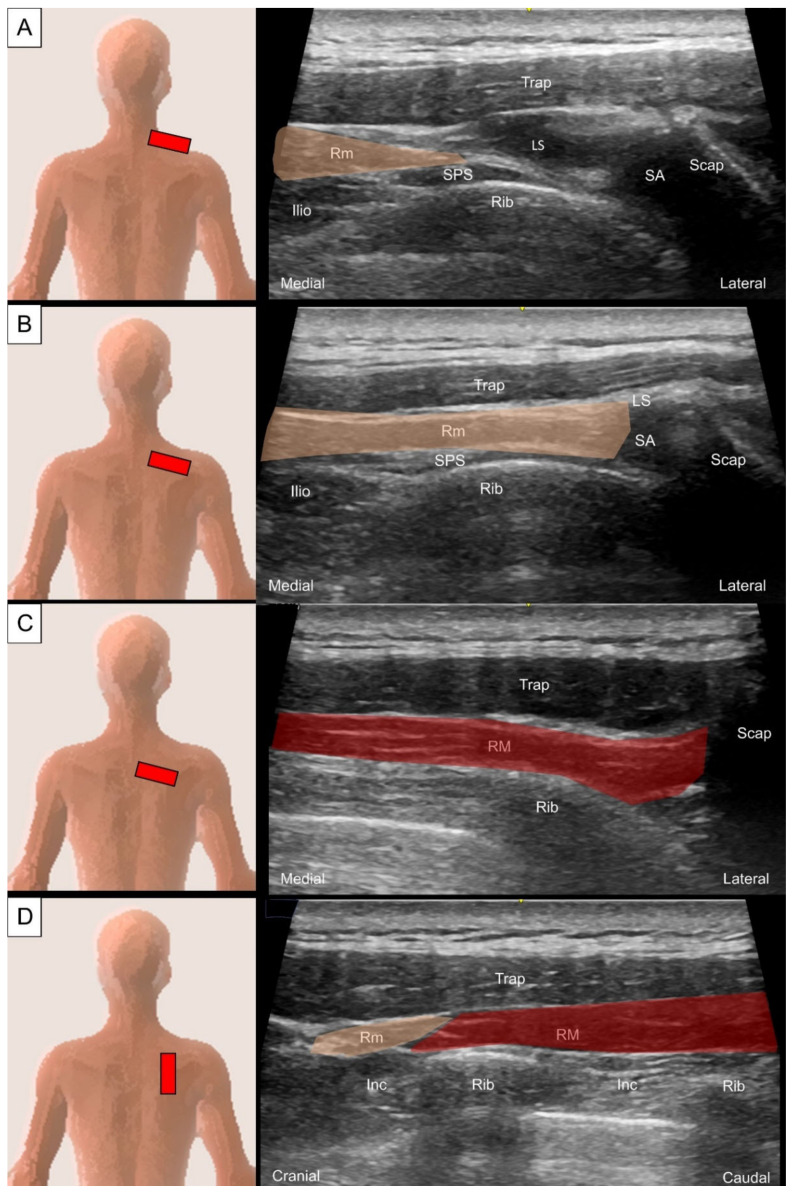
Ultrasound imaging of the rhomboid minor (Rm, skin color shade) at the (A) more craniomedial and (B) more caudolateral aspect, and (C) rhomboid major (RM, red color shade) in the horizontal oblique plane; ultrasound imagining of the rhomboid muscle group (D) in the sagittal plane. Tra, trapezius; SPS, serratus posterior superior; LS, levator scapulae; SA, serratus anterior; Scap, scapula; Inc, intercostal muscle.
